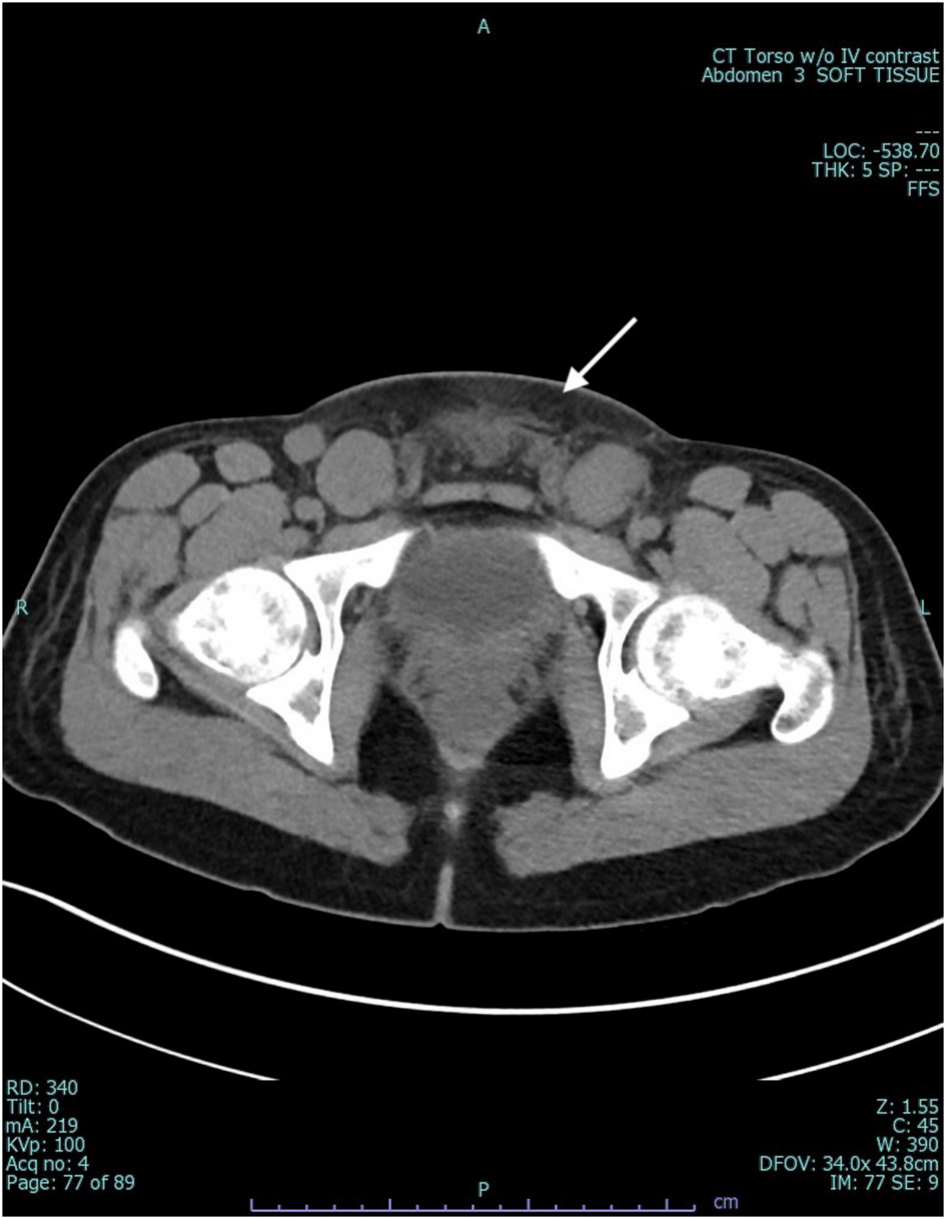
CT pelvis at admission demonstrating bilateral inguinal lymphadenopathy and subcutaneous fat stranding of lower abdominal wall.
